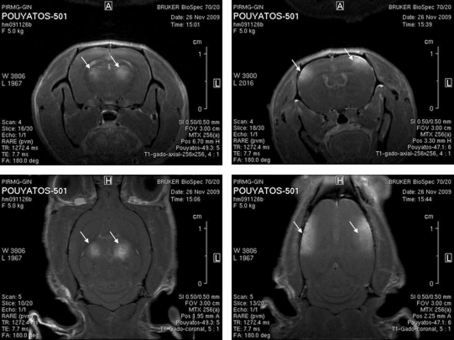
MR imaging of the targeted brain regions in GAERS. T_1_-weighted images acquired 5 min after intravenous injection. Gd-DOTA injection of the evolution of the radio-induced lesion at day 15 after irradiation. The thalamus and the somatosensory cortex were precisely targeted using our alignment procedures (arrows).
